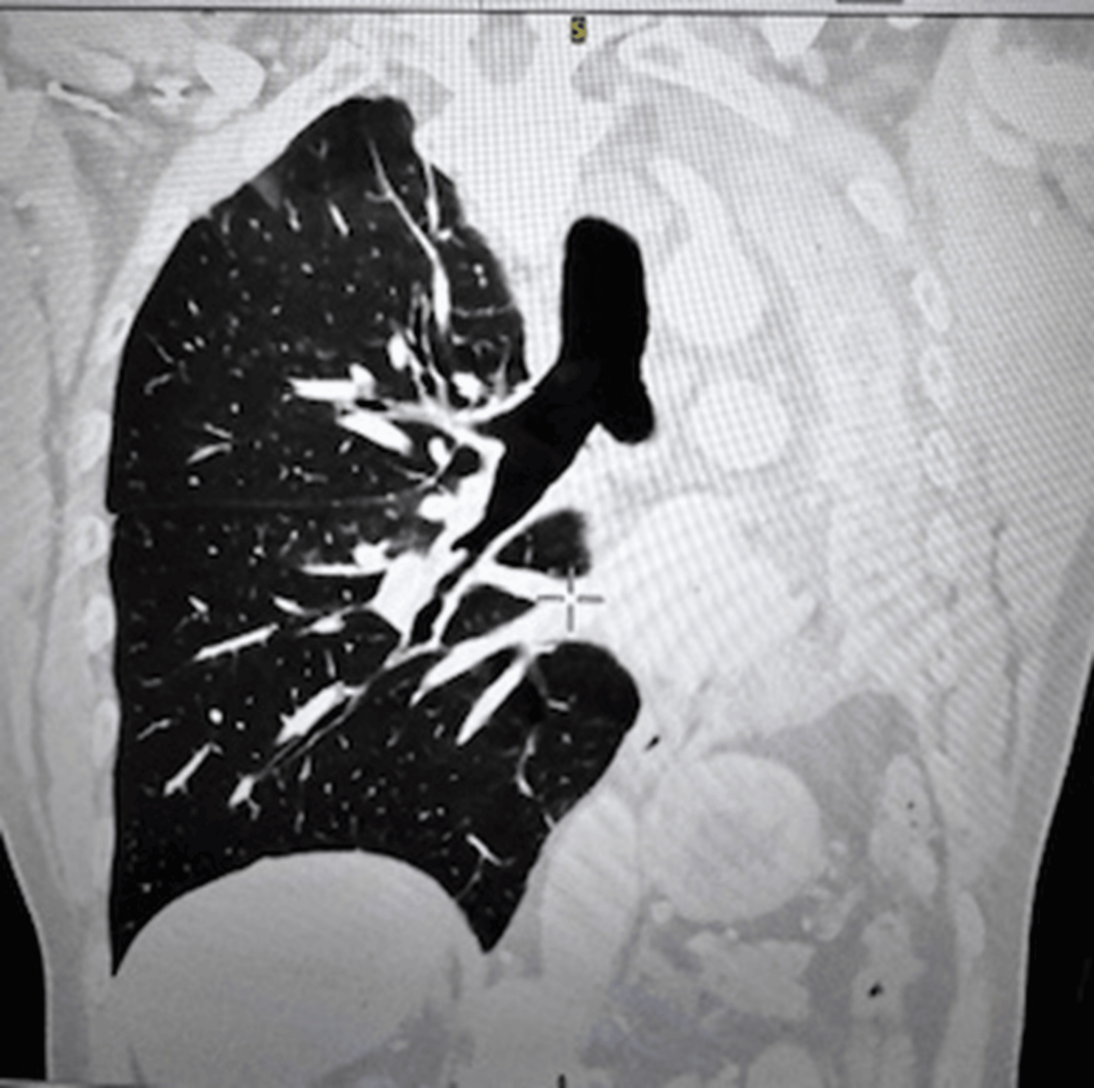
CT thorax in the coronal section showing complete obstruction in the left main bronchus and complete left lung collapse with hyperinflation of right lung and mediastinal shift to the left with left rib crowding.
